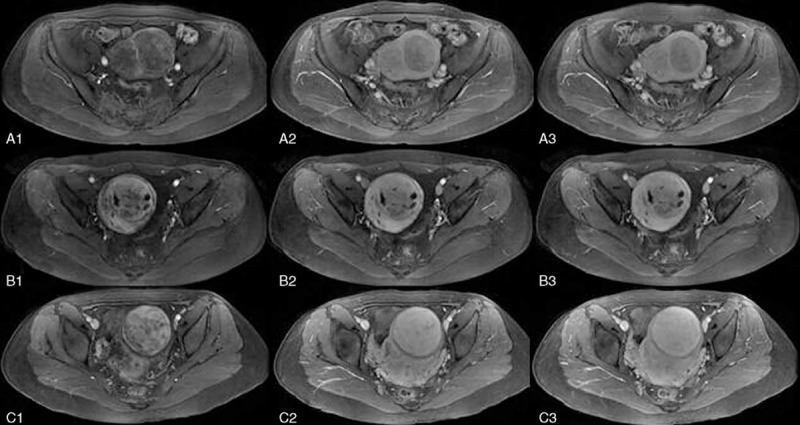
Dynamic contrast-enhanced axial T1-weight magnetic resonance imaging before treatment: A1–A3, B1–B3, and C1–C3 are images acquired at 20, 40, and 60 seconds after contrast injection (from left to right), respectively. (A) Fibroid shows slight enhancement; (B) fibroid shows irregular enhancement; and (C) fibroid shows progressive enhancement.
